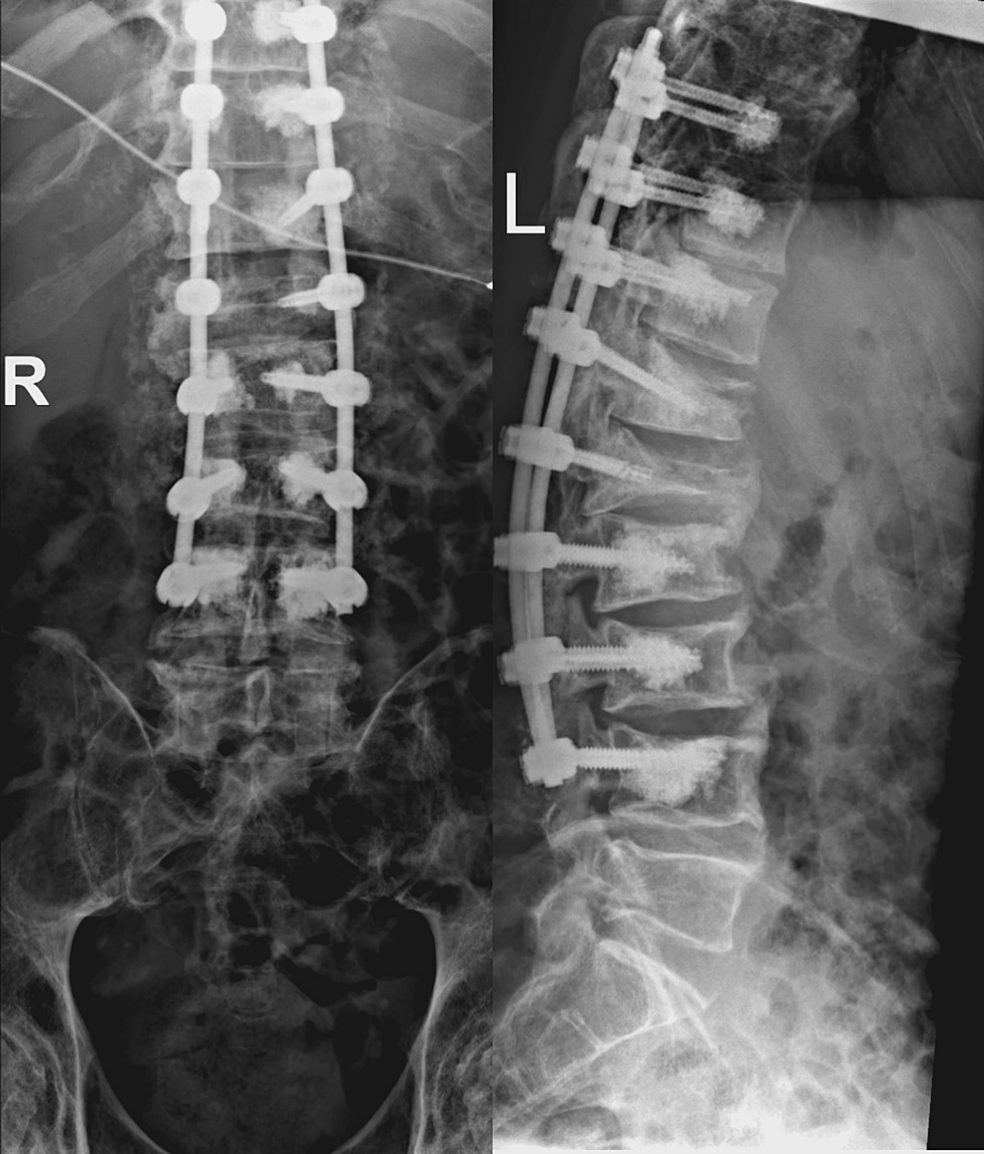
Post-operative X-rays show well-seated T9-L4 posterolateral instrumentation with cement augmentation.
